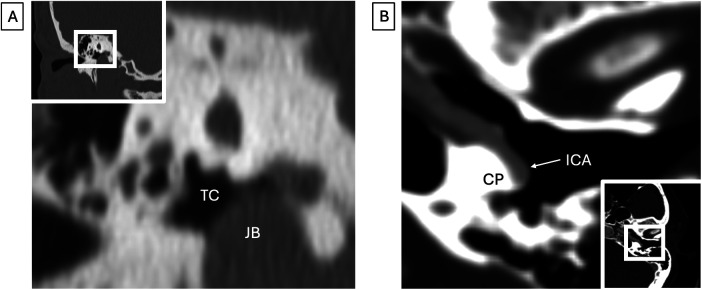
Carotid and jugular anatomy (both images represent different patients). (A) Jugular bulb variation. Coronal CT shows a dehiscent high-riding jugular bulb (JB) protruding into the tympanic cavity (TC). (B) Carotid artery variation. Axial CTA shows an aberrant left internal carotid artery (ICA) coursing across the cochlear promontory (CP).
